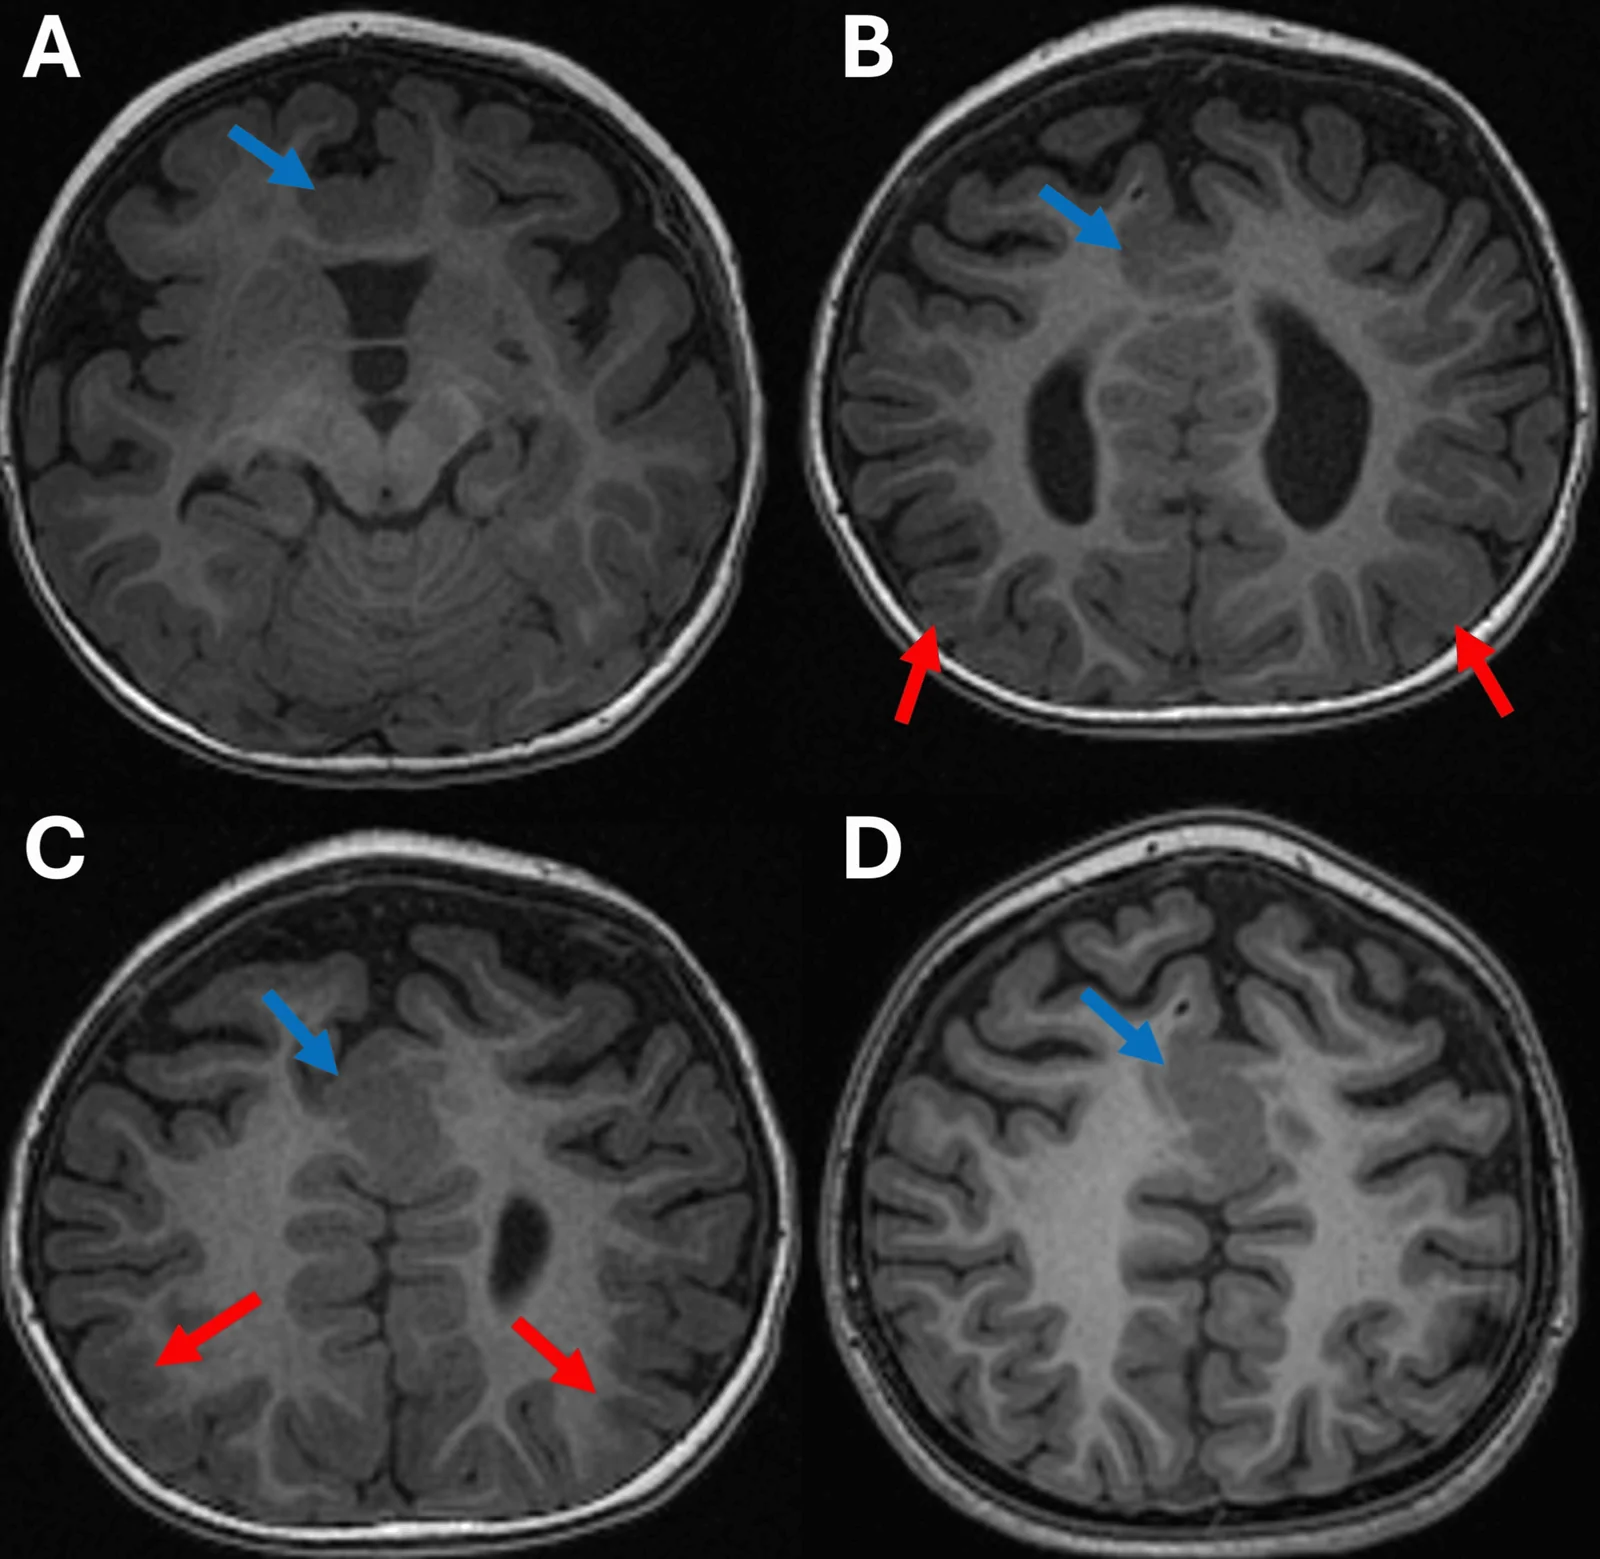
MRI images demonstrating midline fusion of the posterior parts of the frontal lobes and polymicrogyria Axial T1-weighted MRI images without intravenous contrast (A, D) show polymicrogyria (blue arrows) at abnormal fusion of the posterior parts of the frontal lobes. Axial T1-weighted MRI images without intravenous contrast (B, C) show polymicrogyria (blue arrows) at abnormal fusion of the posterior parts of the frontal lobes as well as abnormal sulcations and polymicrogyria bilaterally (red arrows).
